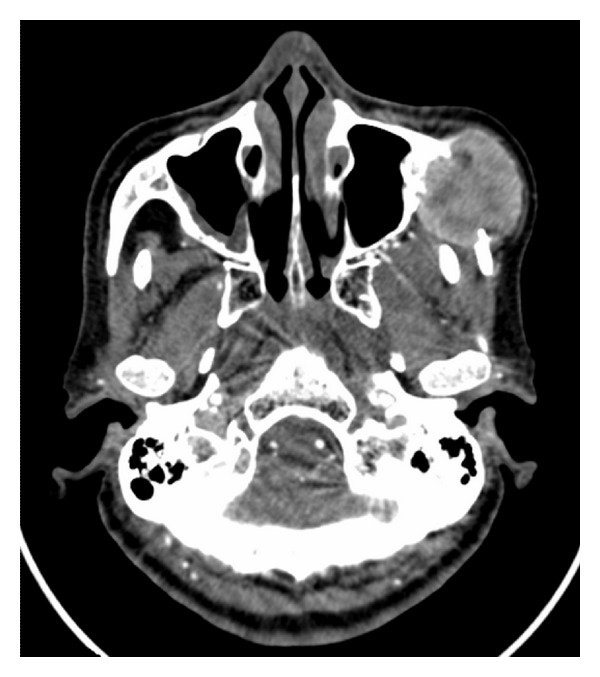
CT of the face with contrast that showed a large enhancing soft tissue mass involving the left malar bone with maxillary wall extension.
